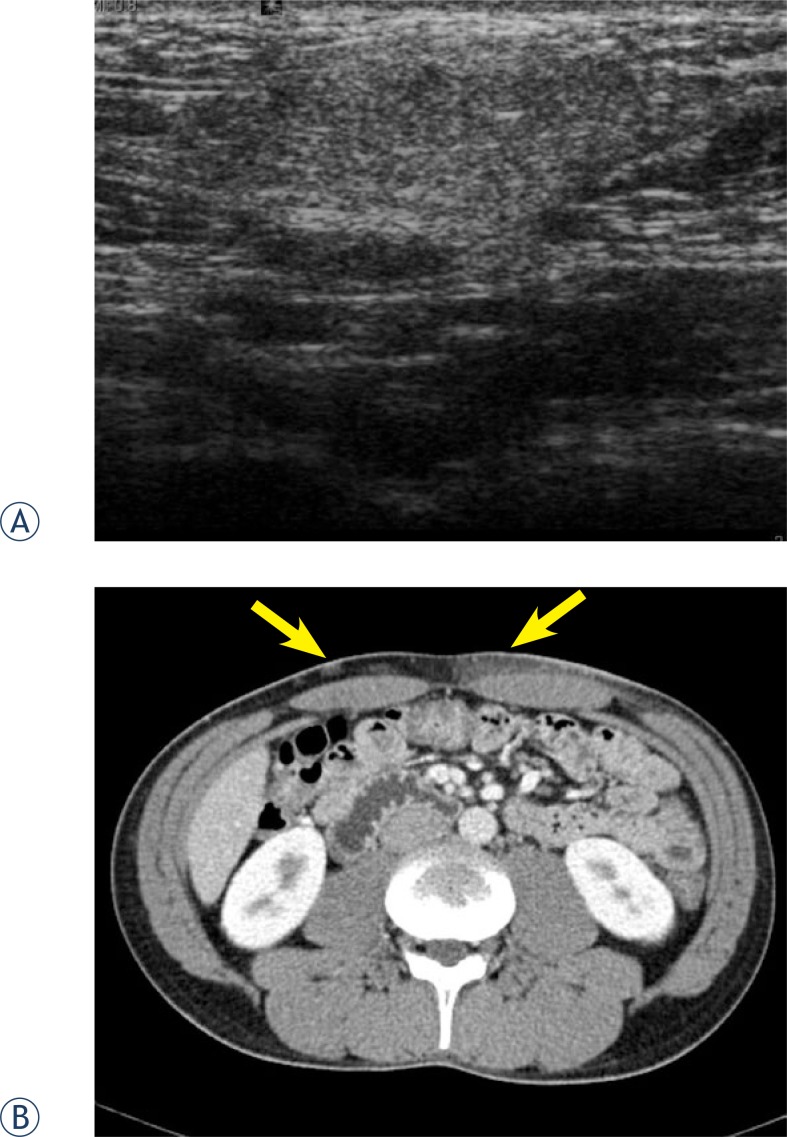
On gray-scale US examination of the lesion in the left abdomen shows ill-defined hyperechoic infiltration (A). Contrast enhanced CT shows multiple mild enhancing nodular and diffuse infiltrative lesions (arrows) in the subcutaneous layer of the anterior abdomen (B).
